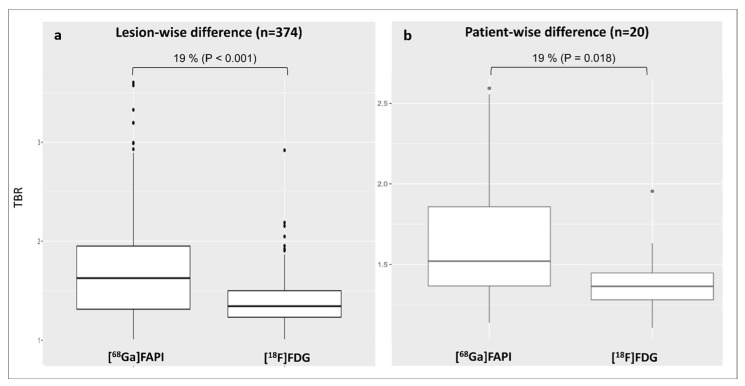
Distribution of arterial lesional TBR values for both tracers according to the per-lesion analysis (a) and patient-based analysis (b). [^68^Ga]Ga-FAPI, [^68^Ga]-labeled fibroblast activation protein inhibitor; [^18^F]FDG, 2-deoxy-2-[^18^F]fluoro-D-glucose; TBR, target-to-background ratio.
